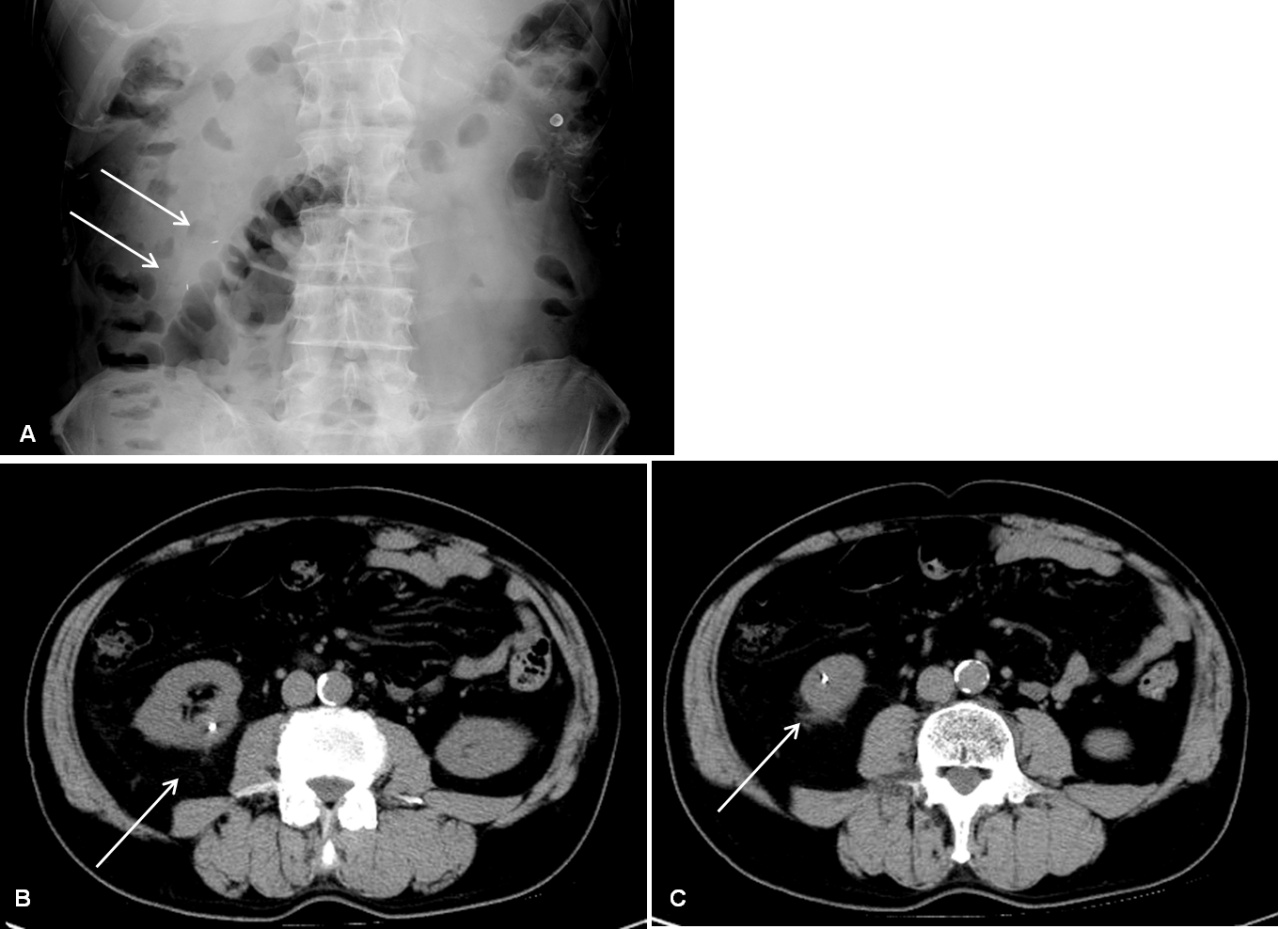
Case 2: Migration of two seeds to the same right kidney. Two weeks after seed implantation, a follow-up abdominal radiograph showed that two seeds had migrated to the right side of the middle abdomen (solid arrows) (A). Subsequent computed tomography showed that these two seeds had migrated to the same right kidney (solid arrows) (B,C).
